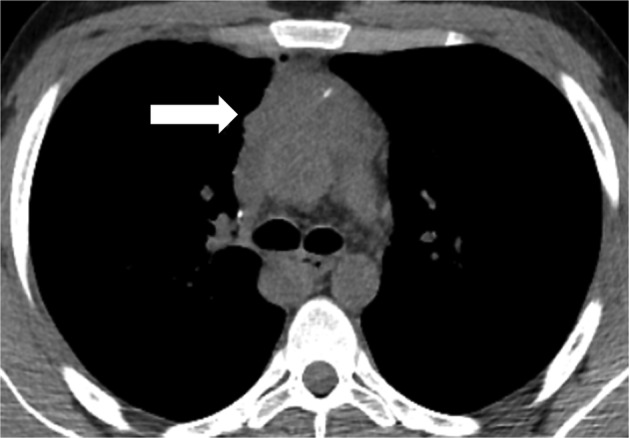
Axial view of CT scan evaluation performed after four cycles of chemotherapy according to BEP protocol. Partial response (PR) per RECIST 1.1 was reached with a significant decrease of tumor mass (arrow) and a reduction in main bronchus compression.
